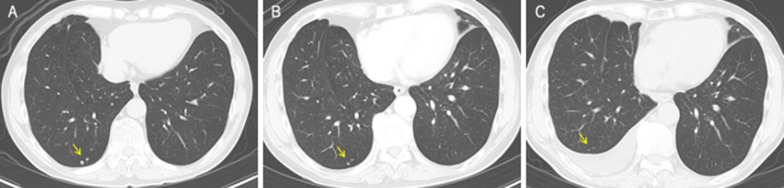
Chest CT findings. a CT at the presentation of the patient showing pulmonary nodules in the right lower lobe of the lung suspected to be lung metastasis (arrow). b The size of nodules decreased after 2-week administration of lenvatinib (arrow). c The size decreased further 3 months after the hepatectomy
